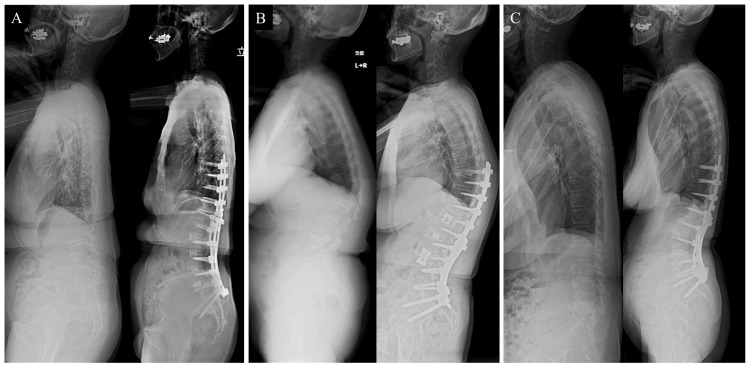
Lateral X-ray images of the representative cases before and after surgery (A) First-stage lateral lumbar interbody fusion was performed from L2/3, L3/4, and L4/5. One week later, second-stage posterior corrective instrumented fusion surgery was performed from T9 to the pelvis. (B) First-stage posterior corrective instrumented fusion surgery was performed from T8 to the pelvis. One week later, second-stage anterior cage insertion with lateral interbody fusion was performed. (C) First-stage L2/3 lateral lumbar interbody fusion was performed. One week later, second-stage L4 pedicle subtraction osteotomy (grade 3) and posterior corrective fusion surgery from T10 to the pelvis were performed.
